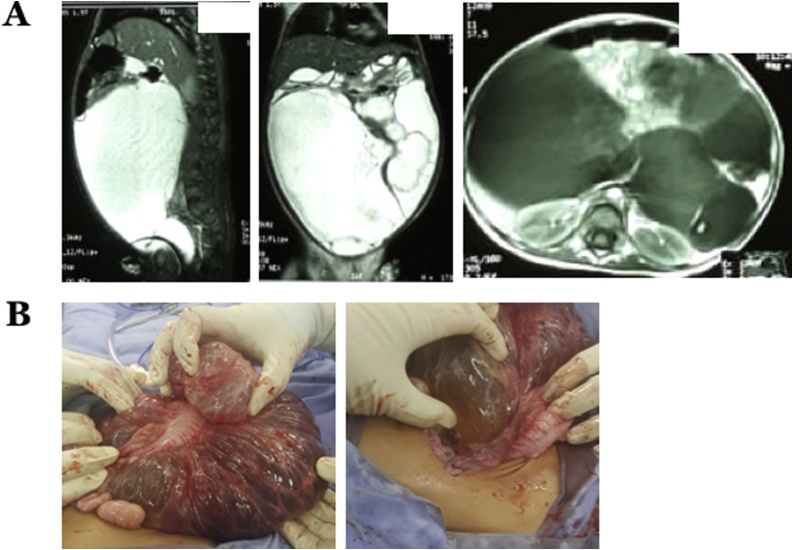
A) Contrast magnetic resonance imaging revealed suspicious multilocular large mesenteric cysts. B) Intraoperative findings showed multiple mesenteric cysts attached to the transverse colon and descending colon.
